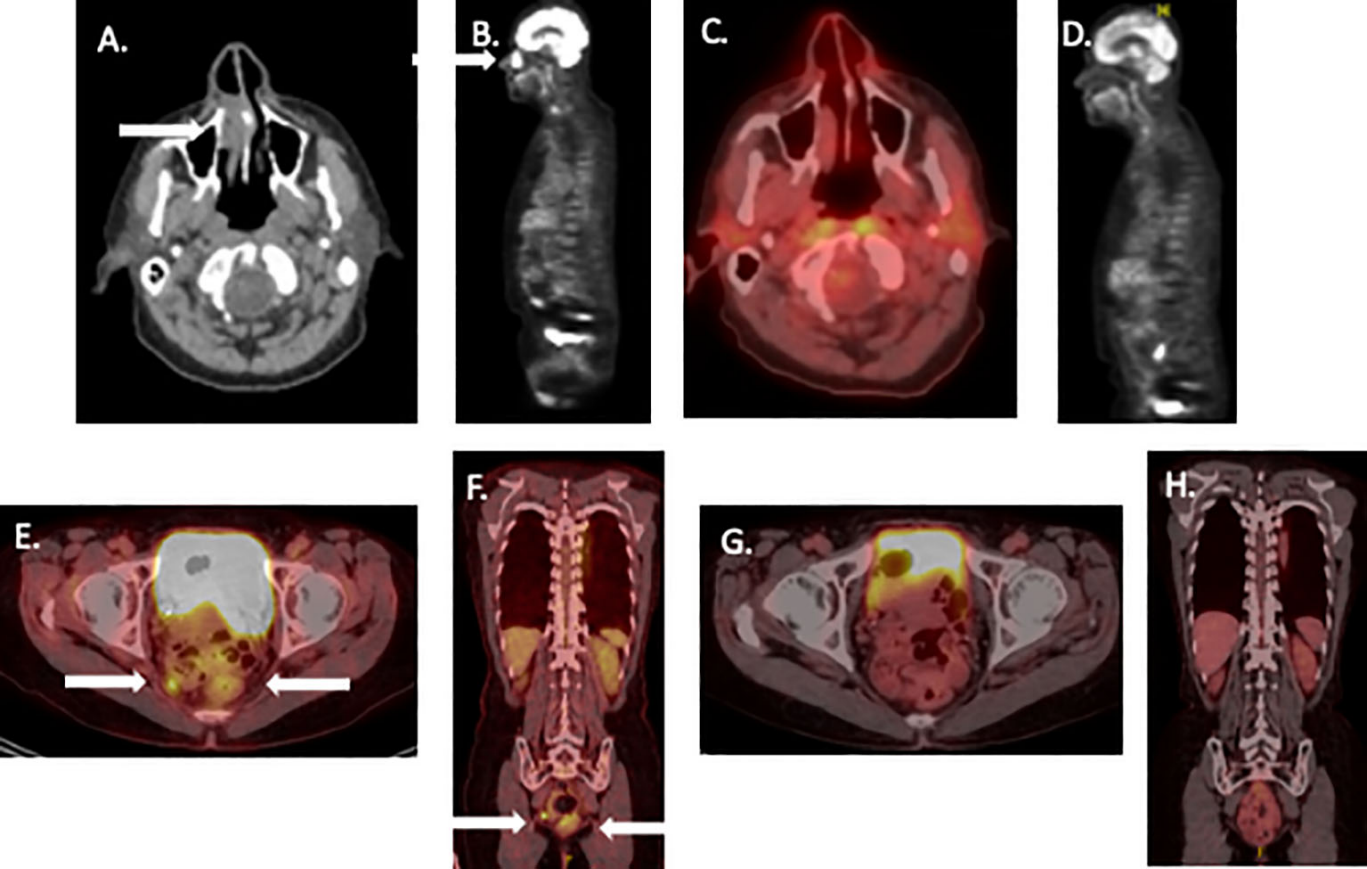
Pre- and post-treatment scans of patients undergoing neoadjuvant therapy for mucosal melanoma. (A, B): Pretreatment PET/CT scan images patient 1 showing nasal melanoma. (C, D): similar PET/CT views 3 years later. (E, F): Pretreatment PET CT of patient 2, (G, H) similar PET/CT views showing complete remission 2 years later. White arrows show tumor site on initial images.
